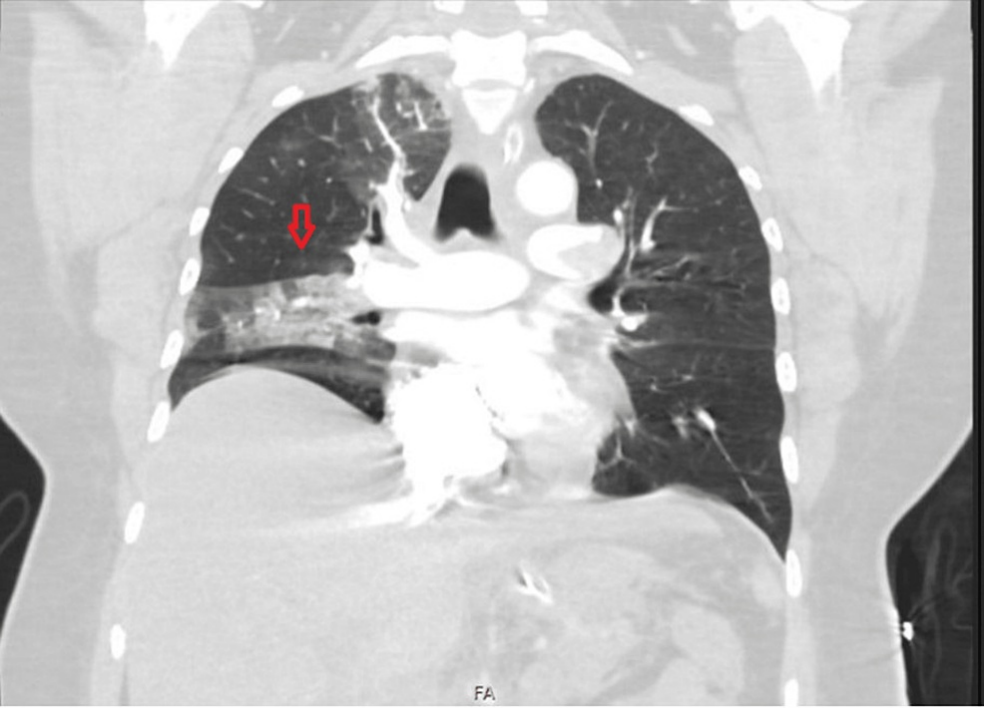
CT-pulmonary angiography: coronal view showing pulmonary emboli with segmental pulmonary infarcts in the right lower lobe
